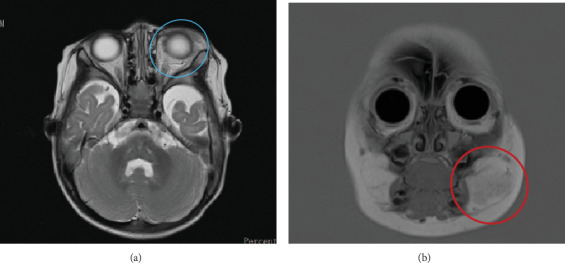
(a) Axial view of the brain MRI taken 7 months after the patient started oral propranolol, indicating a smaller left periorbital mass (blue circle). (b) The coronal view of the brain MRI showed a less defined subcutaneous mass in the left cheek region (red circle).
